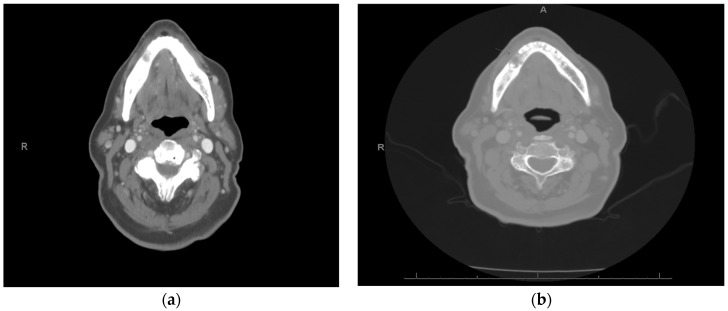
Restaging imaging (a) in soft tissue window of axial CT scan shows evidence of interval decrease in size of the right buccal thickening and symmetry with contralateral buccal region, while bone window (b) shows similar appearance of mandibular erosion performed approximately 3 months after treatment initiation.
